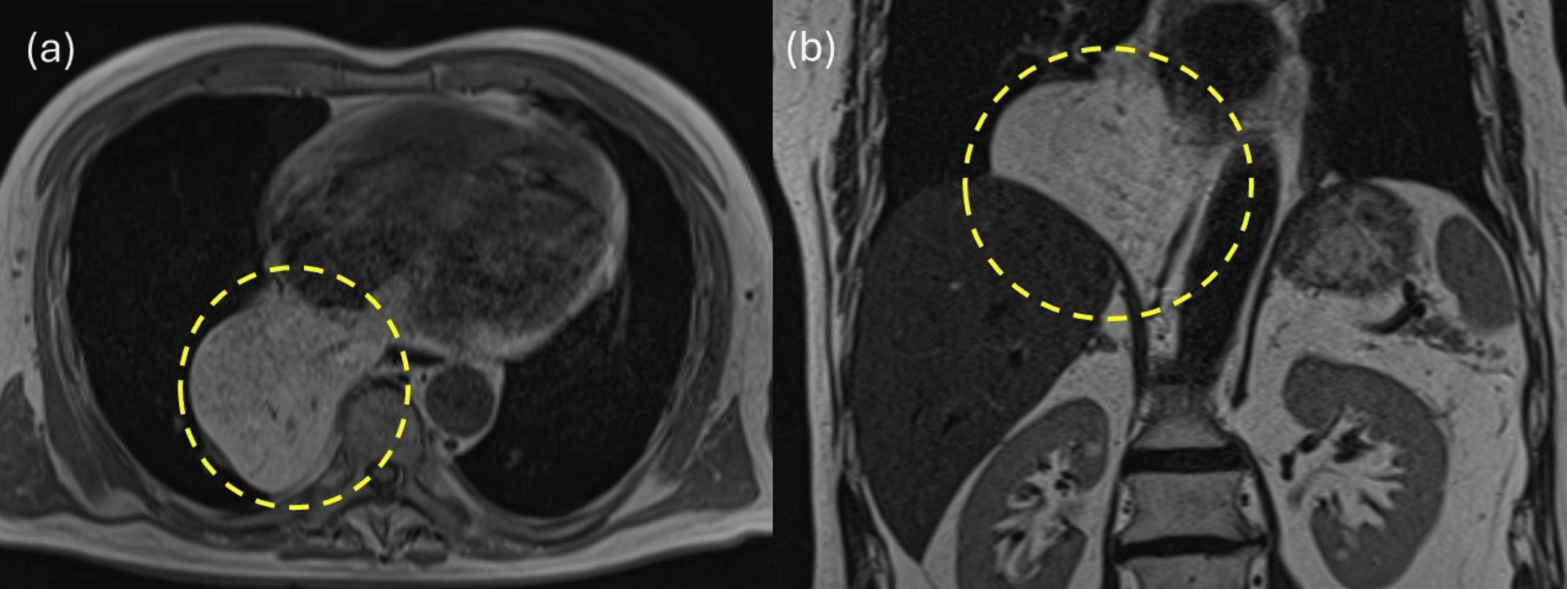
Contrast-enhanced MRI Prolapse of the intraabdominal fat into the mediastinum via the esophageal hiatus
